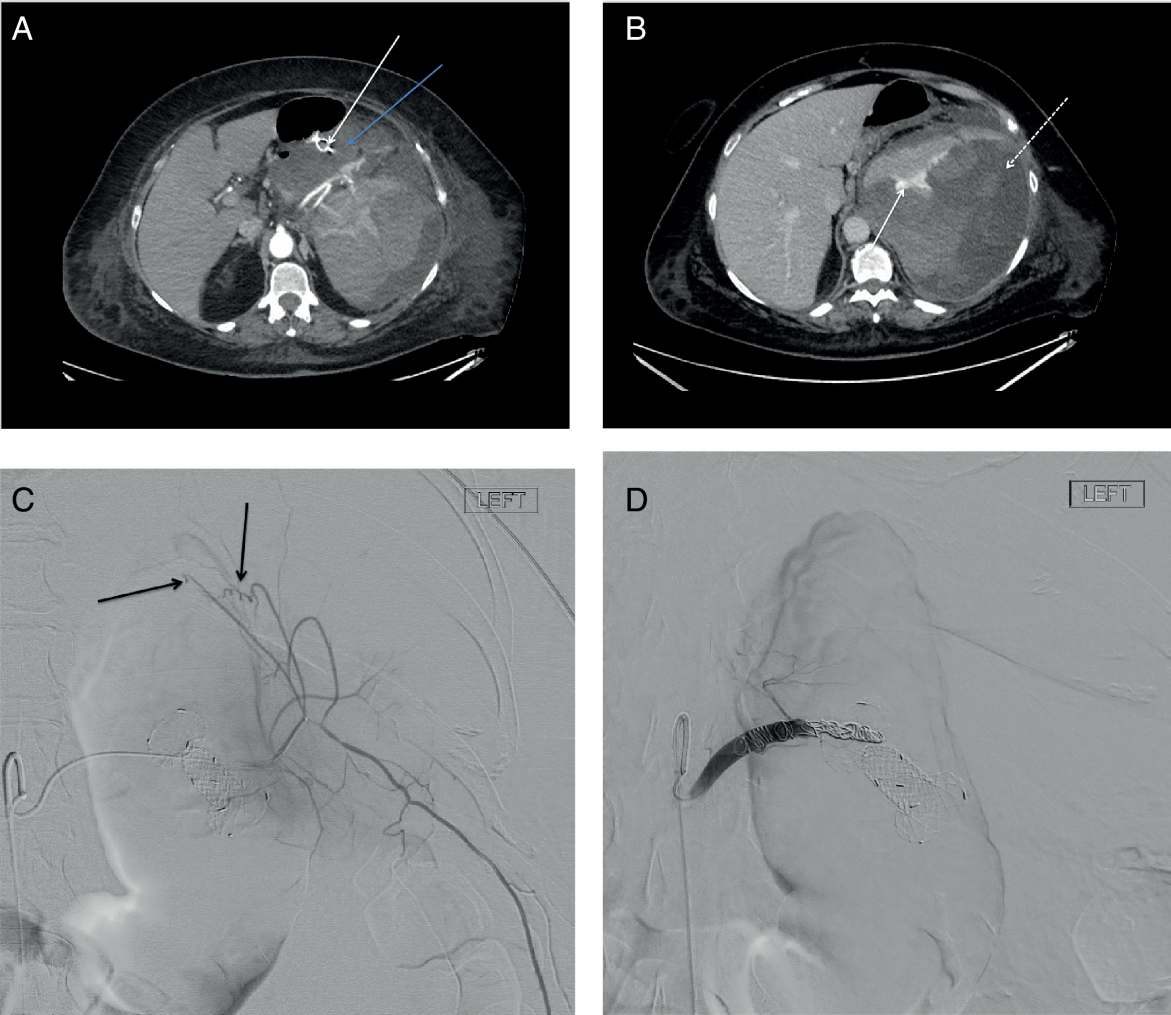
Radiographic images of pseudocyst drain and angiography of bleeding site. (A) Computerized tomography (CT) abdomen: blue arrow—multiple large fluid collections, largest 9.8 x 11.4 x 8.7 cm in the pancreas tail region; white arrow—4 cm x 12 mm lumen-apposing metal stent (Hanaro Stent, M. I. Tech, Korea) placed for drainage, (B) CT abdomen with contrast: solid arrow—fluid collection measuring 12 cm with the accumulation of extravasated contrast suggesting hemorrhagic transformation; dashed arrow—large splenic subcapsular/pericapsular hematoma, (C) arteriogram revealing abnormal distal capsular splenic branches with no active extravasation and (D) angiogram of the splenic artery showing proximal embolization with platinum coils.
